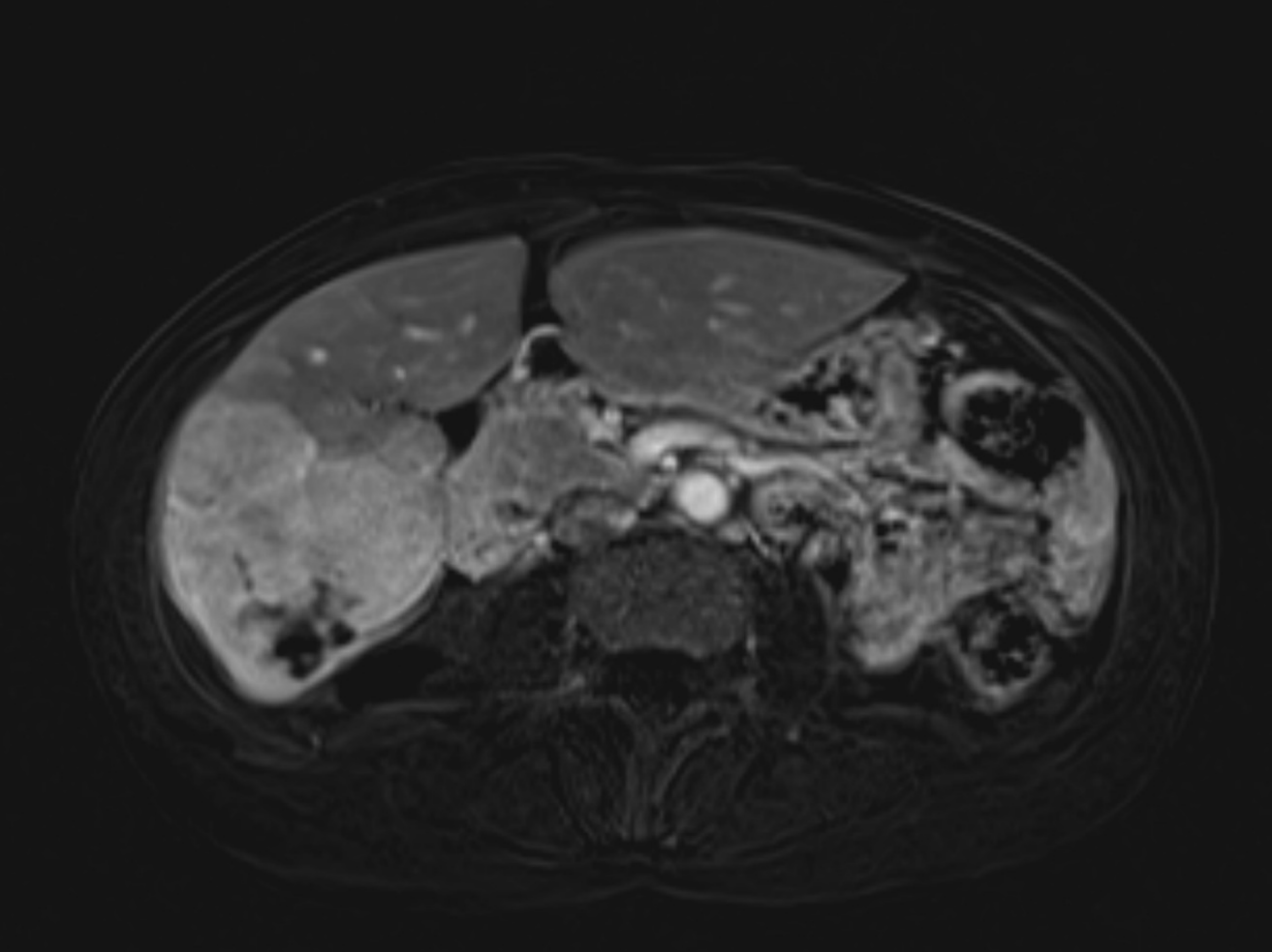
Representative axial slice from MRI obtained after first SIRT procedure
